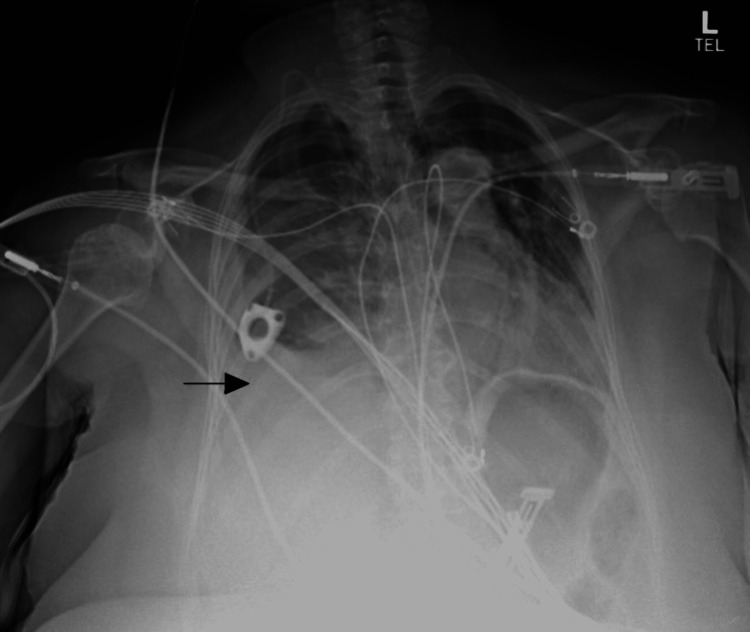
Second postoperative chest radiograph The image shows the right internal jugular mediport again seen with the tip at the level of atriocaval junction. The arrow designates a new small-to-moderate size right pleural effusion/hemothorax.
